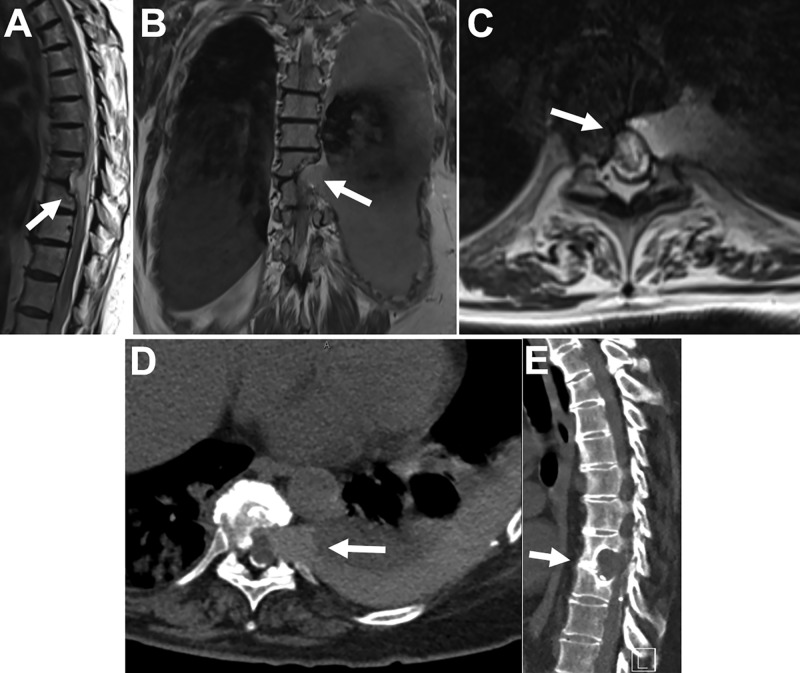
Preoperative MRI and CT imaging of the thoracic spine (A) sagittal T2-weighted MRI demonstrating a ventrolateral defect at the left T8-9 level (arrow). (B) coronal T2-weighted MRI showing herniation of the pleural cavity into the surgical defect (arrow) and the presence of a pleural effusion. (C) axial T2-weighted MRI showing displacement of the ventral spinal cord into the defect and contact of the herniated pleura with the thecal sac contents (arrow). (D) axial CT myelogram demonstrating a hyperdense fluid extending from the spinal canal to adjacent pleural space consistent with a dural-pleural fistula (arrow). (E) sagittal CT image of the thoracic spine demonstrating the osseous defect (arrow)
